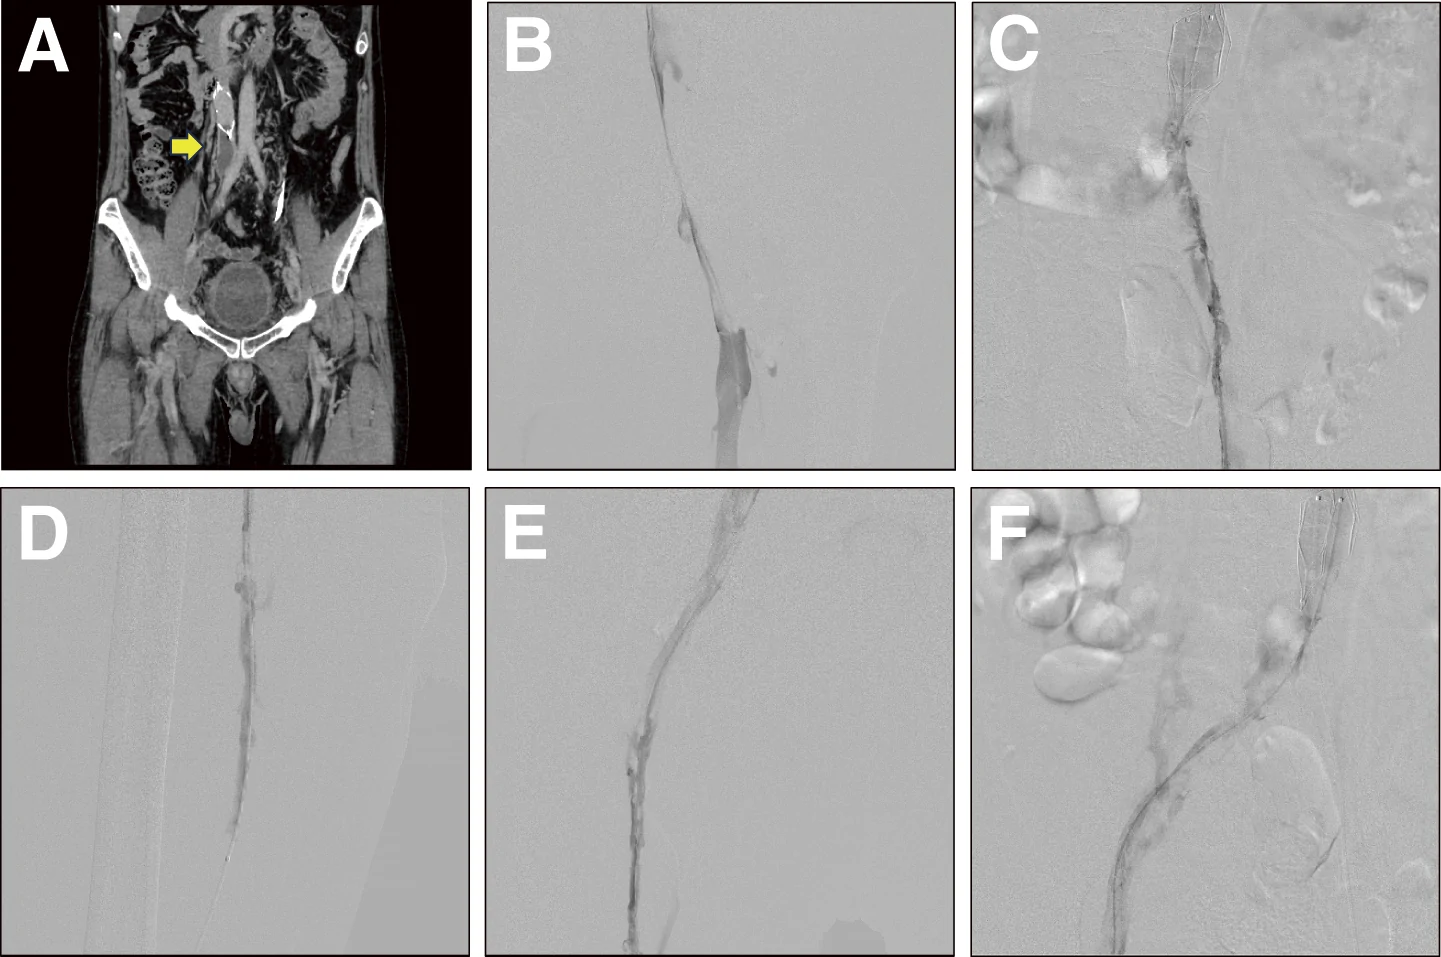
A CT shows thrombus formation in the IVC just below the IVCF (A). A venography through a Fountain catheter. Each venography shows a large amount of thrombus in the right proximal femoral vein to the right iliac vein (B, C), and in the left distal femoral vein to the left iliac vein (D–F). The IVC below the IVCF contains a large amount of thrombus. Because of the prone position, (B) and (C) show the right leg vein, and (D–F) show the left leg vein. IVC, inferior vena cava; IVCF, inferior vena cava filter
